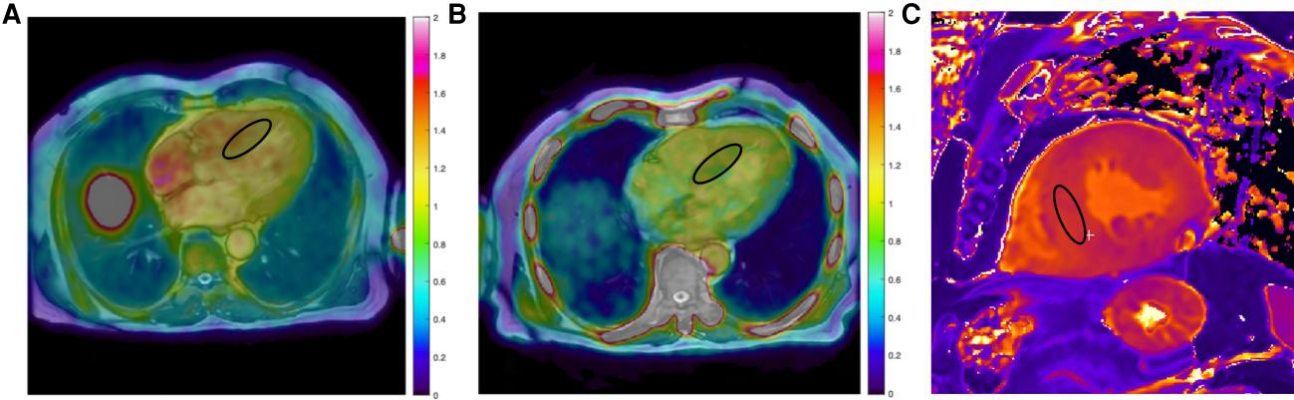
PET-MR images displaying demonstrating (*A* & *B*) myocardial uptake suggestive of amyloid infiltration and microcalcification, and (*C*) native T1 mapping by modified Look-locker inversion recovery (MOLLI) sequence, demonstrating diffusely increased native T1 values.
